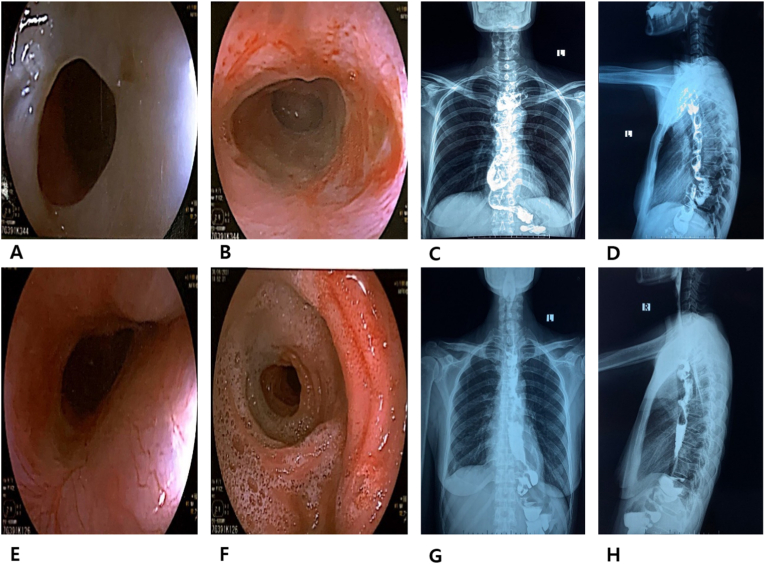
Endoscopic and X-ray images at follow-up examination, 2 months after the surgery. First case: A esophago-ileal anastomosis; B jejunum-colon anastomosis; C, D. Chest X-ray of the esophagus-ileum-colon-jejunum segment with Barium Sulfate (PA and lateral views); Second case: E. esophago-ileal anastomosis; F. Valve Bauhin; G, H. Chest X-ray of the esophagus-ileum-colon-jejunum segment with Barium Sulfate (PA and lateral views).
